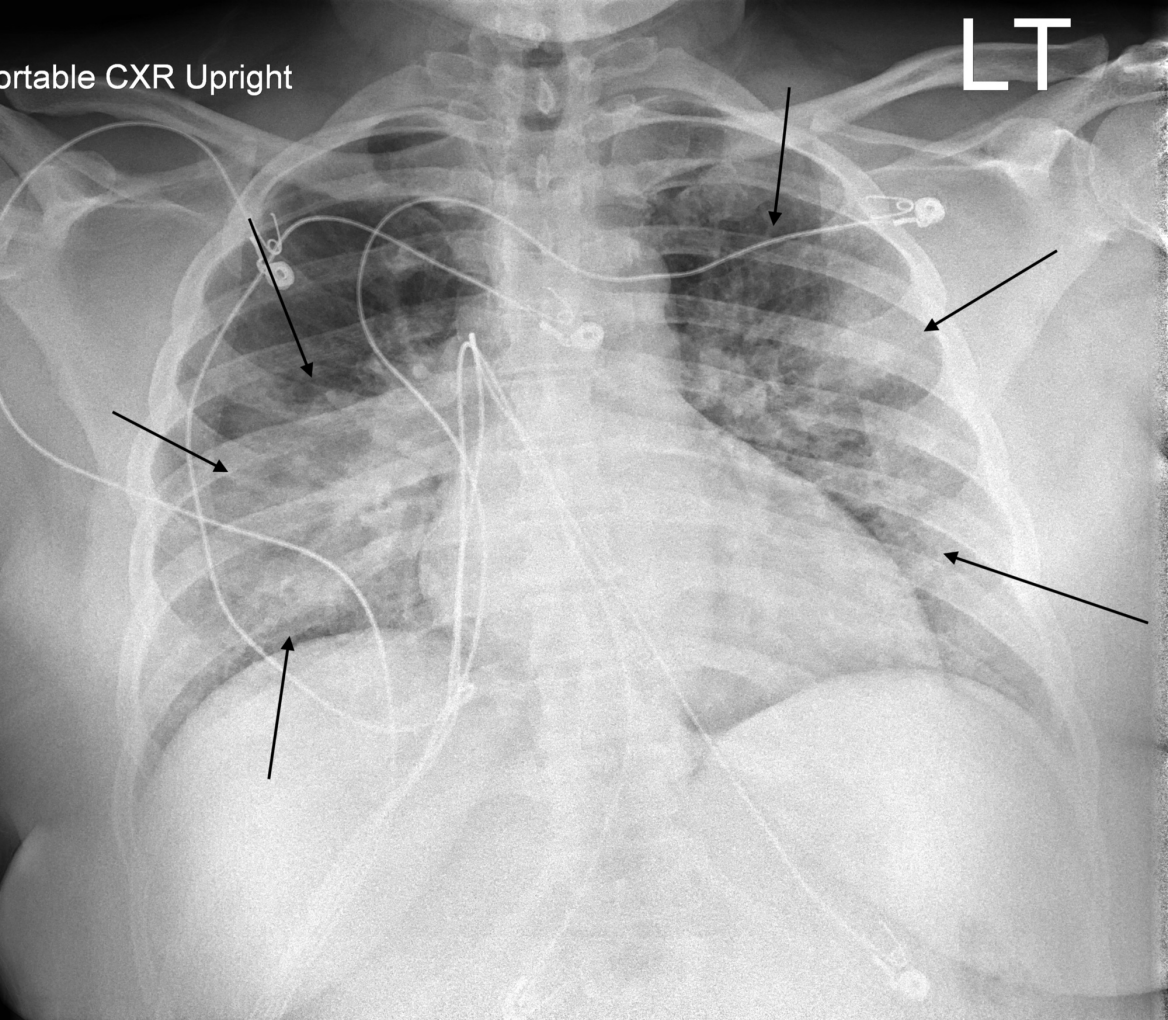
Anteroposterior chest x-ray demonstrating bilateral areas of consolidation consistent with atypical pneumonia
